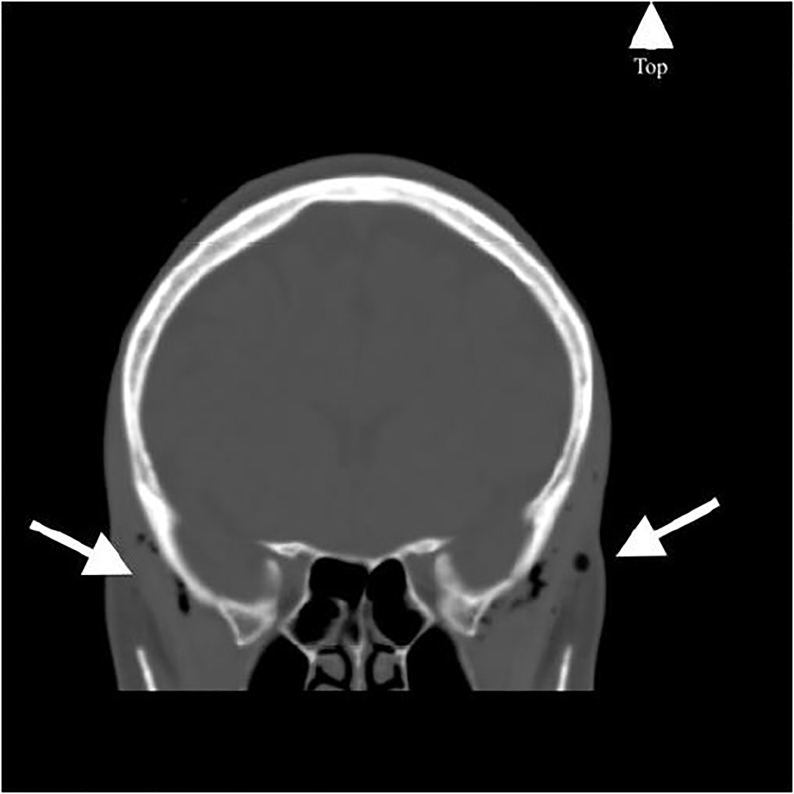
Coronal computed tomography of the brain without contrast demonstrating bilateral air tracking into the subcutaneous temporal tissue (arrows).
